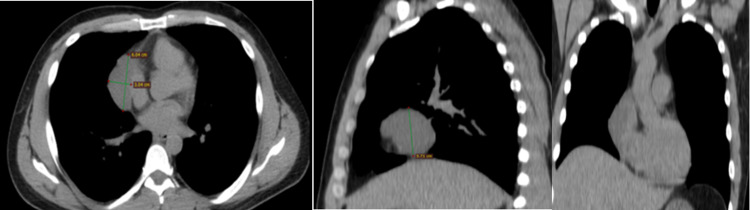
Chest CT demonstrating the pericardial cyst.
